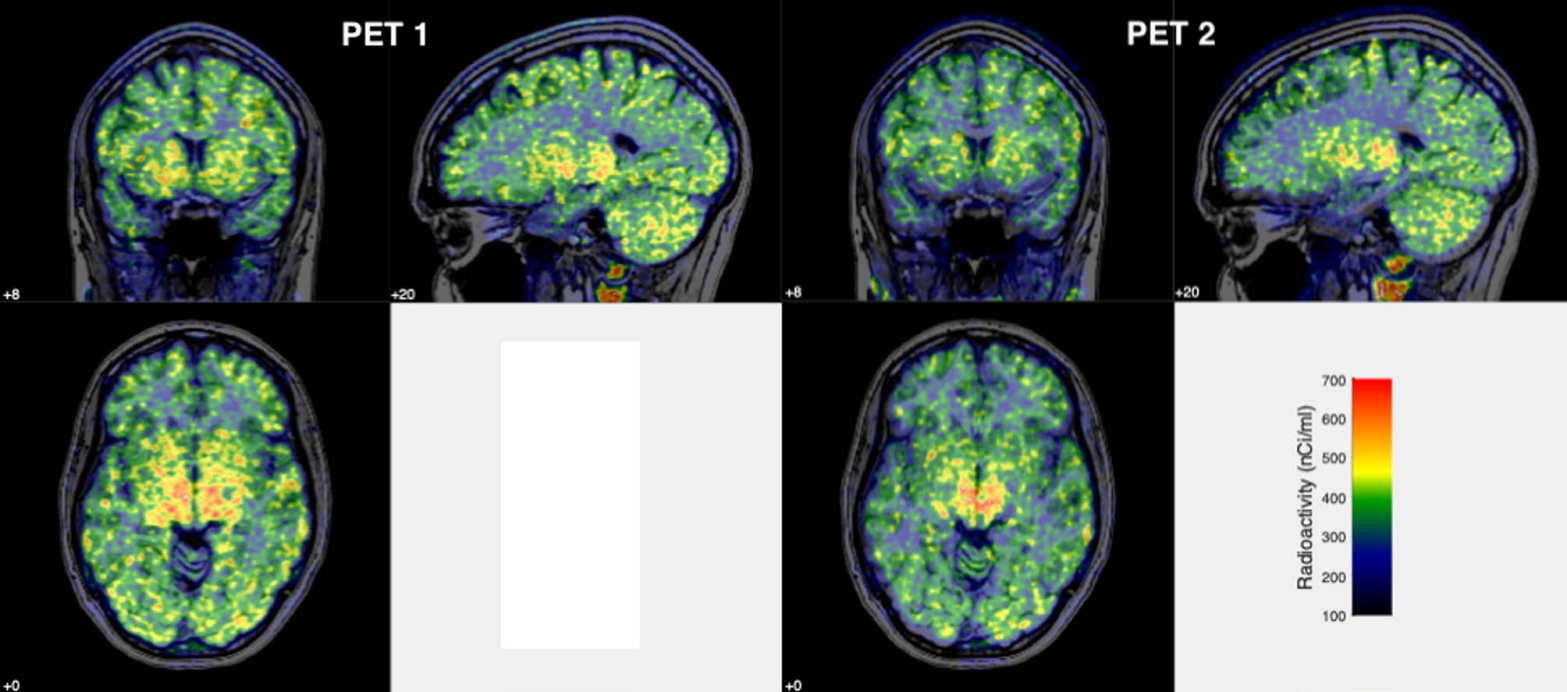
Summation PET images (AUC, 0–30 min), radiographic view, superimposed on corresponding MRI images in a healthy volunteer after i.v. injection of [^11^C]PXT012253, at two different occasions (A and B)
